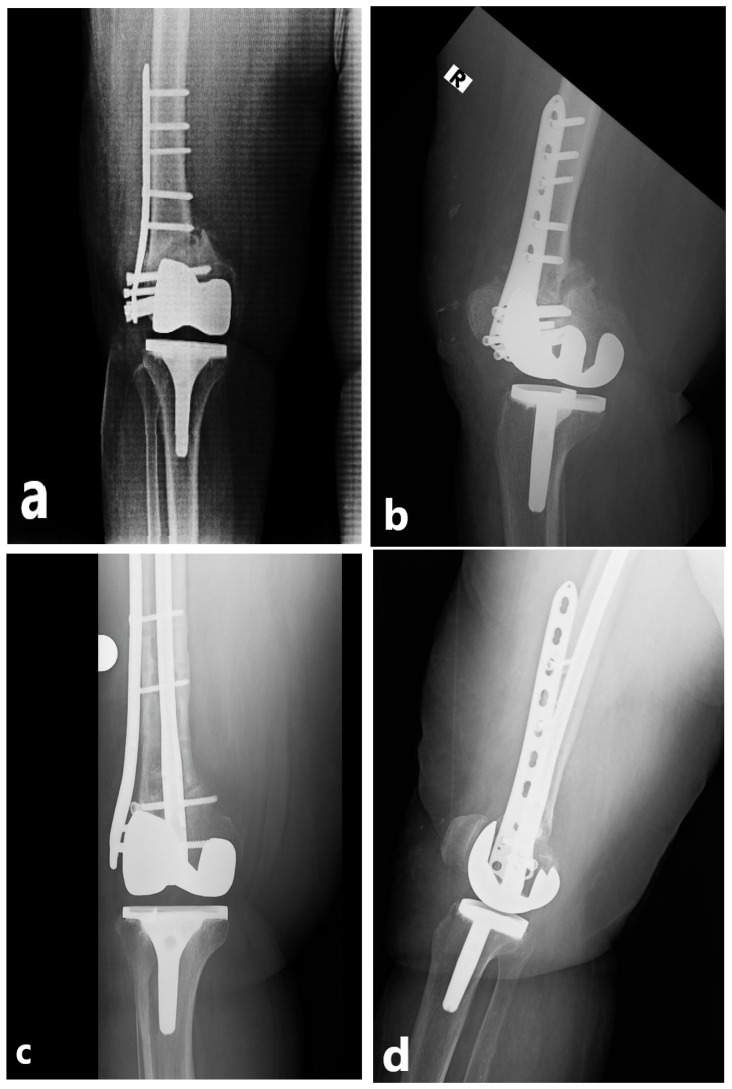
Radiographic images of a 72-year-old female. (a,b) Anteroposterior and lateral views demonstrating implant failure after the initial fixation of a periprosthetic distal femur fracture around a total knee arthroplasty. (c,d) Anteroposterior and lateral radiographs at postoperative 3 months following revision with a nail–plate combination. The radiographic marker “R” indicates the right extremity.
